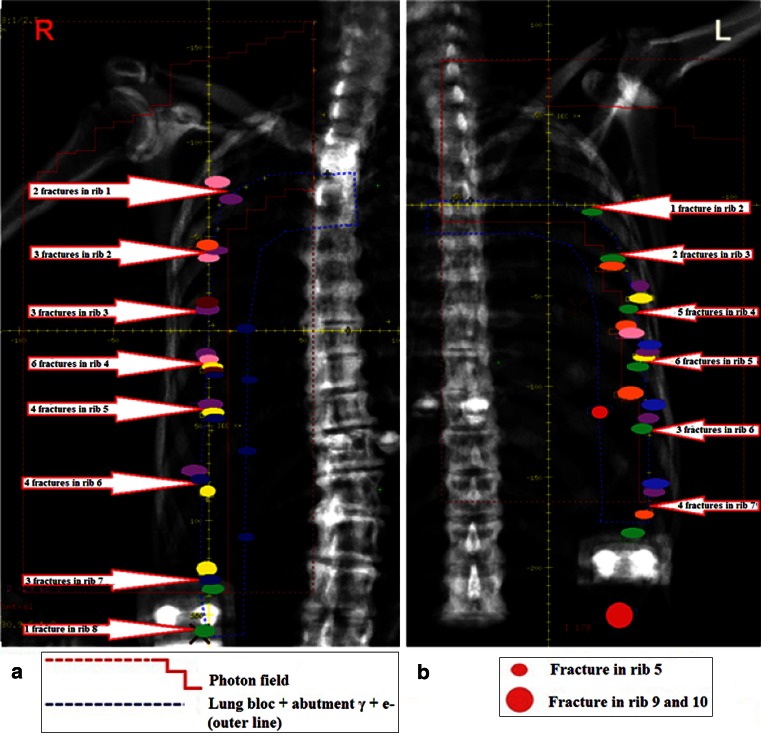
Chest wall CT-sans of two random breast cancer patients showing the AMC treatment plan setup: a Left chest wall. b right chest wall, different patient. Each color represents rib fractures of an individual patient. In red fractures in rib 5, 9 and 10 are indicated for a patient which were not associated with abutment regions.*γ* photon field. *e*
